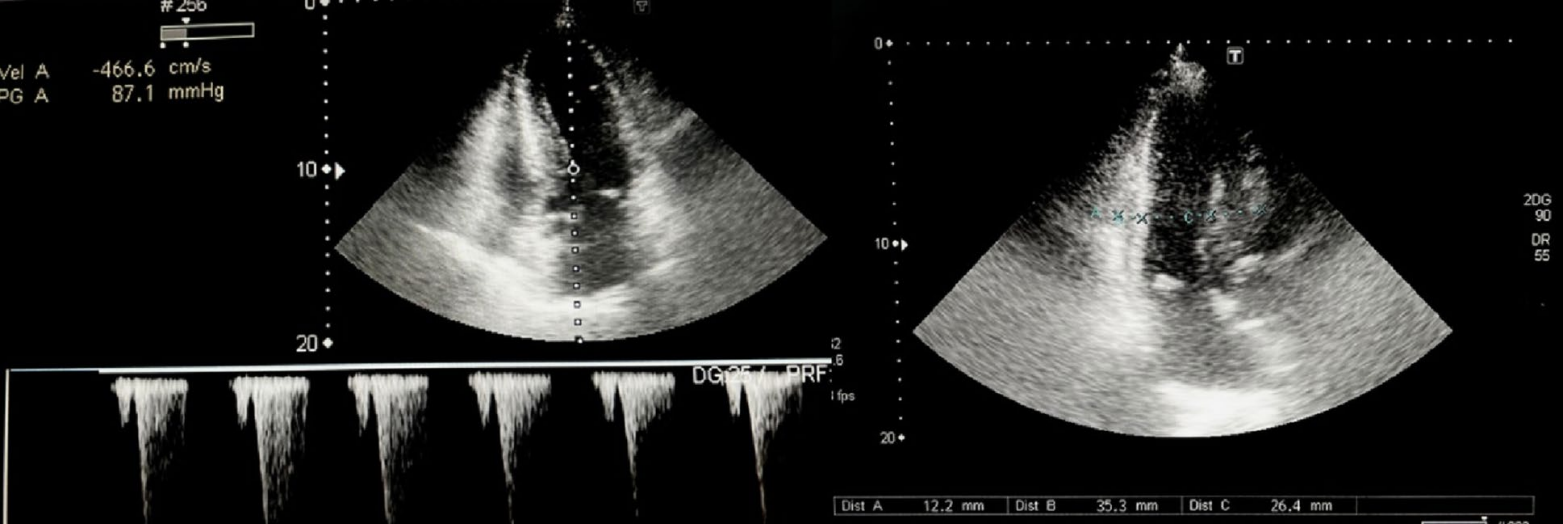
Transthoracic echocardiogram revealed HOCM, septal hypertrophy of 26.4 mm, posterior wall thickness of 12.2 mm, dynamic obstruction of the LVOT, with a maximum velocity of 4.6 m/s and a gradient of 87.1 mmHg. The left ventricular diastolic dimension was 35.3 mm, with a left ventricular ejection fraction (LVEF) of 70%. Mild diastolic dysfunction type 1 was present, along with mild left atrial dilation and no evidence of pulmonary hypertension
